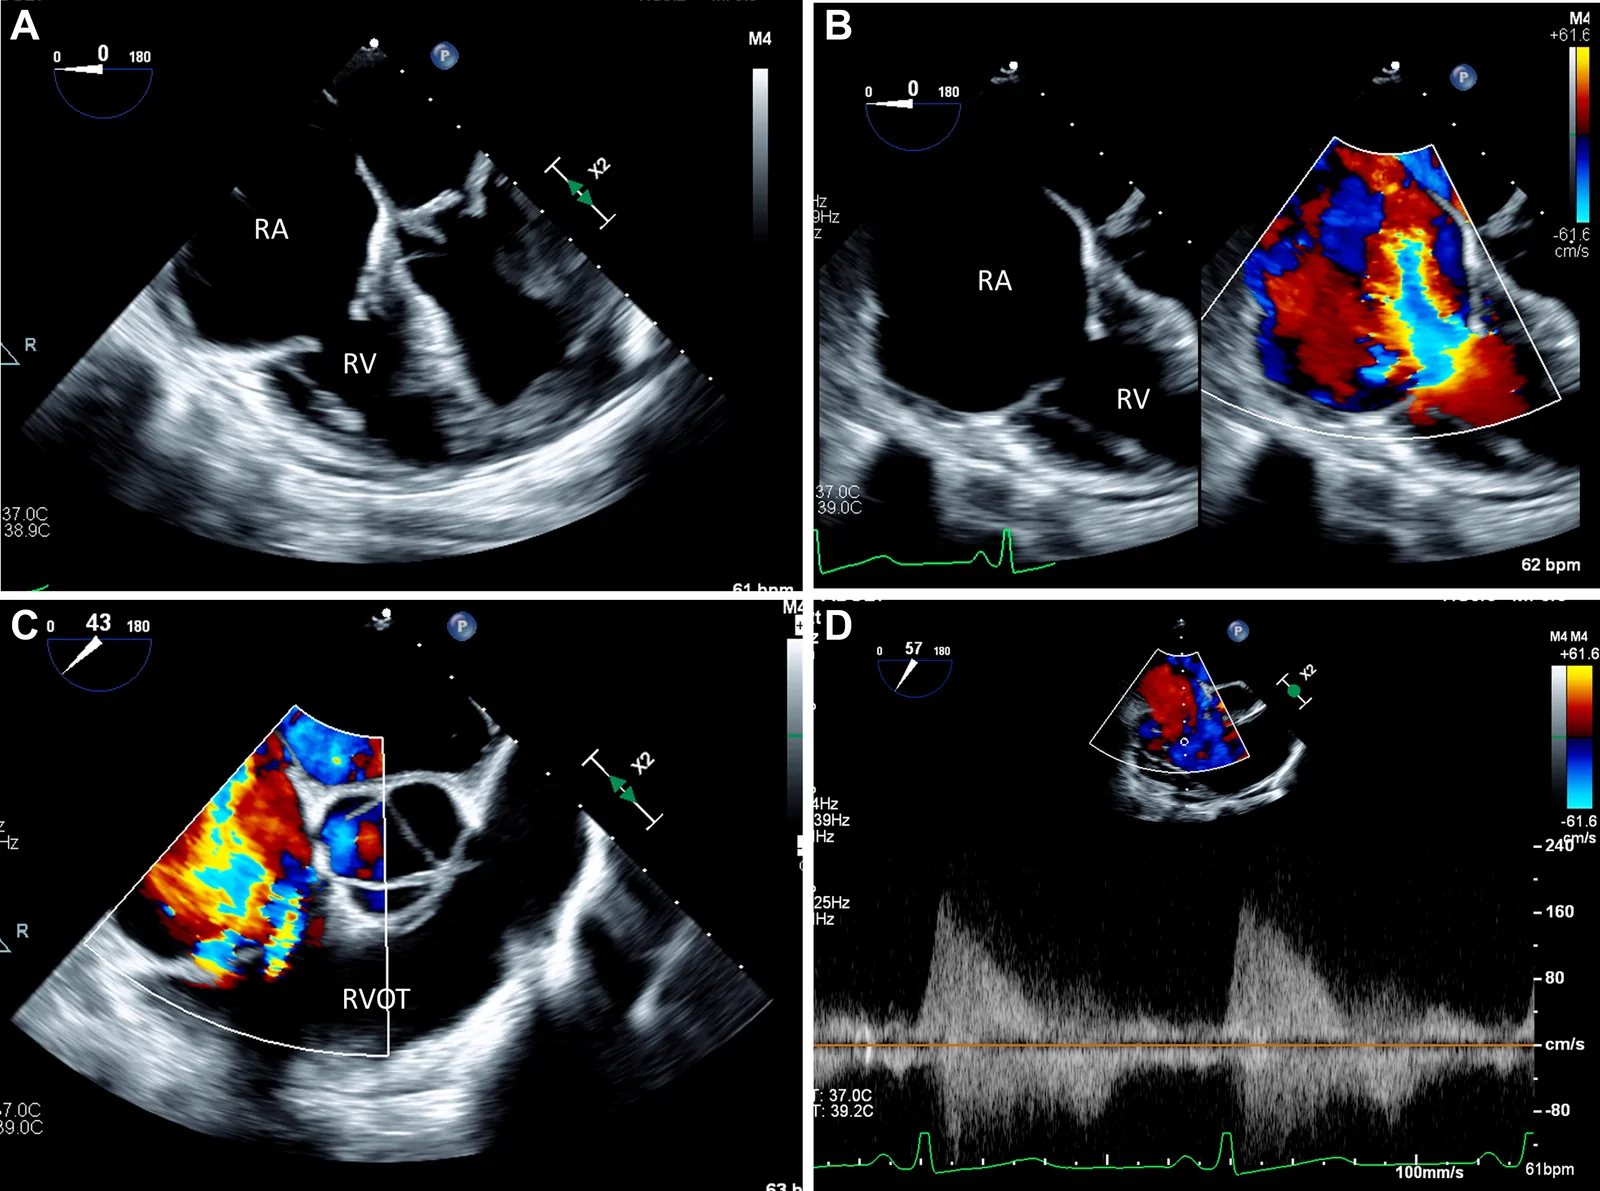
Transesophageal Echocardiography Showing Thickened and Malcoapted Tricuspid Valve With Severe Regurgitation Transesophageal echocardiography midesophageal view showing thickened, retracted, and severe malcoaptation of tricuspid valve (A) with resultant severe tricuspid regurgitation (B, C). Continuous-wave Doppler with dense triangular shape jet with rapid systolic decline characteristic of severe tricuspid regurgitation (D). RA = right atrium; RV = right ventricle; RVOT = right ventricular outflow tract.
